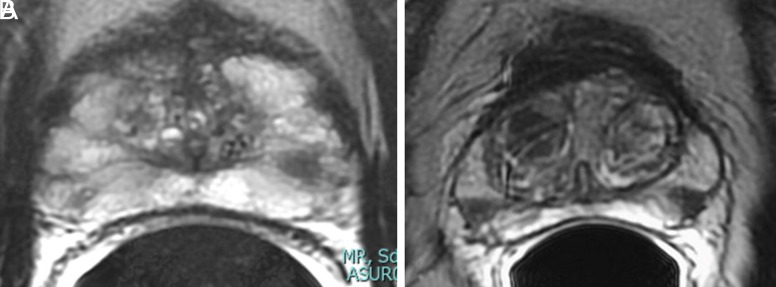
Magnetic resonance images of axial sequences T2WI (A, B) of tumor infiltration of the neurovascular bundle. Nodular lesion far (A) and close (B) to the neurovascular bundle.
